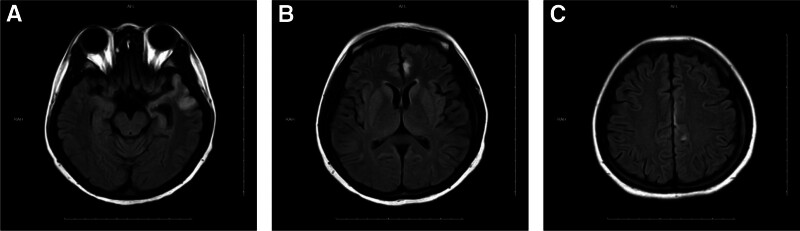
(A–C) December 7, 2023 – MRI demonstrates patchy T2/FLAIR hyperintensities in the left temporal, frontal, and parietal lobes. MRI = magnetic resonance imaging.
